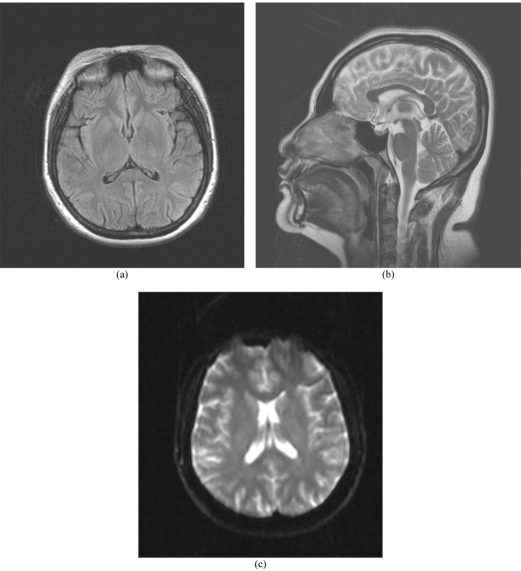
Follow up MR imaging performed 8 weeks after discharge with (a) axial FLAIR, (b) sagittal T2-weighted and (c) axial ADC map showing complete resolution of the SCC lesion and normalization of ADC values.
